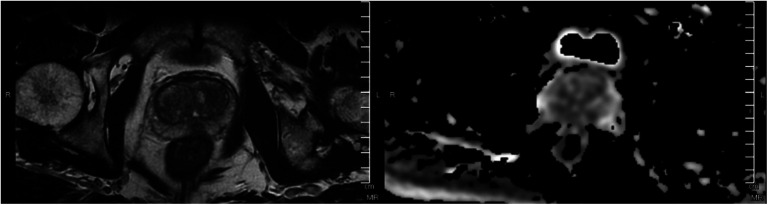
MRI images used for Fusion biopsy. T2W (T-2 weighted imaging) images and ADC (Apparent diffusion coefficient) images show a left paramedian peripheral zone lesion. Eleven systematic biopsies were negative. A total of 1/5 targeted biopsies showed 4 + 4 PCa. He went to have a radical prostatectomy which showed Gleason 4 + 3 T3a N0M0 disease. MRI, magnetic resonance imaging; PCa, prostate cancer.
